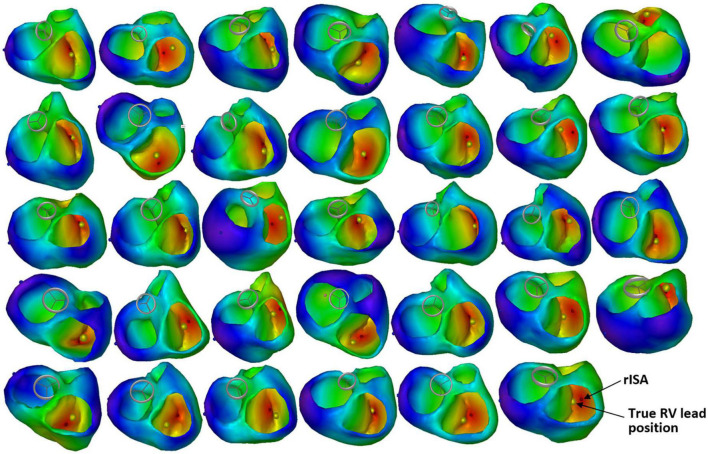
Reconstructed 3D ventricular activation models for all 34 patients. The red marker indicates the reconstructed initial site of activation (rISA), and the yellow marker indicates the true right ventricular (RV) lead position determined by computed tomography (CT).
